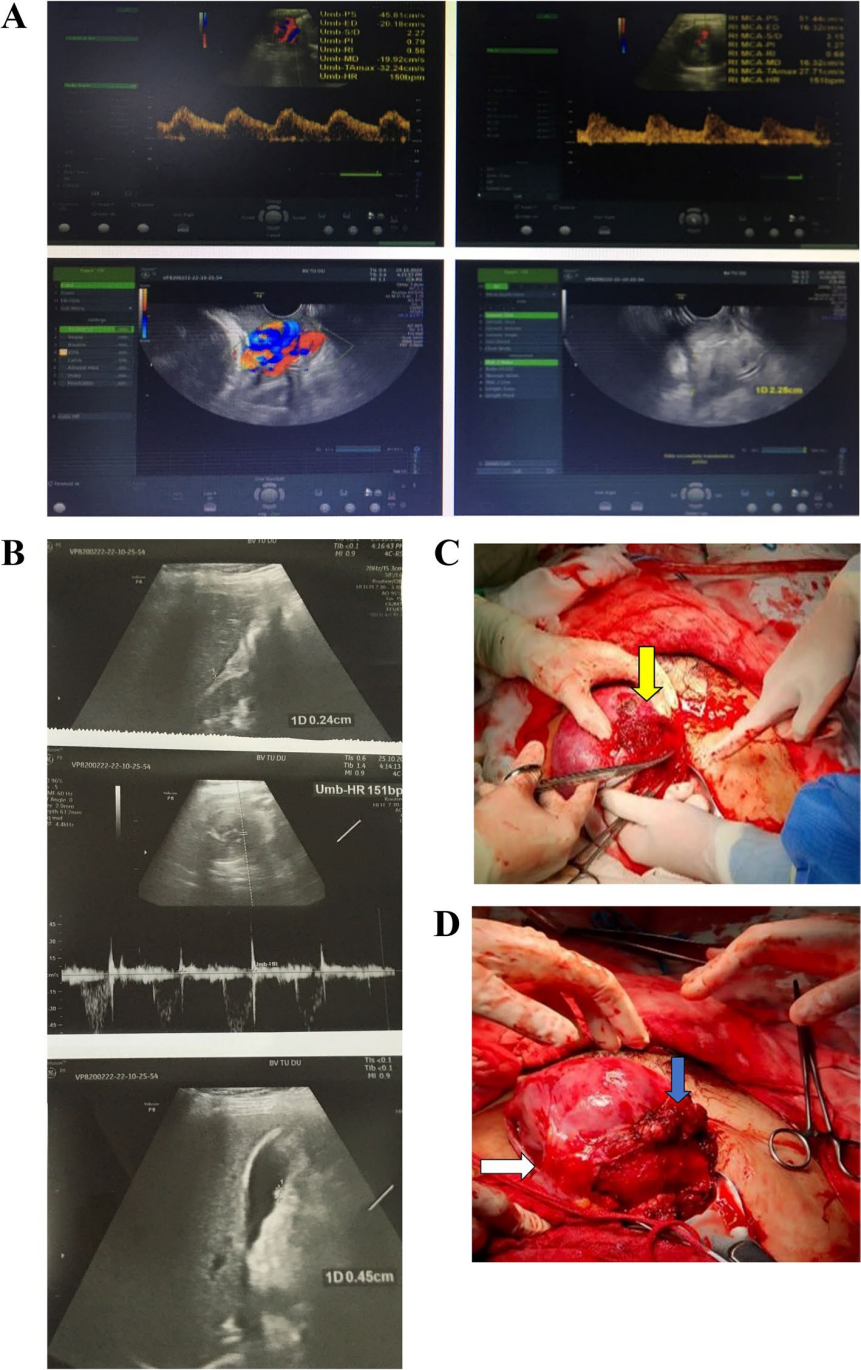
Ultrasonography shows A a single a live fetus and proliferative vasculature at the cervix. B Free fluid collection in the abdominal cavity. C Extravasation in the right adnexa, suspected to arise from the right utero-ovarian plexus (yellow arrow). D Uterine closure accompanied with a multiple hemostatic sutures were performed (blue arrow). One of the adherent bandages existed between the lateral posterior of the uterus and the abdominal anterior wall of the abdomen (white arrow). The bleeding stopped after releasing a part of the adhesion, excision of fragile tissue, and suturing
